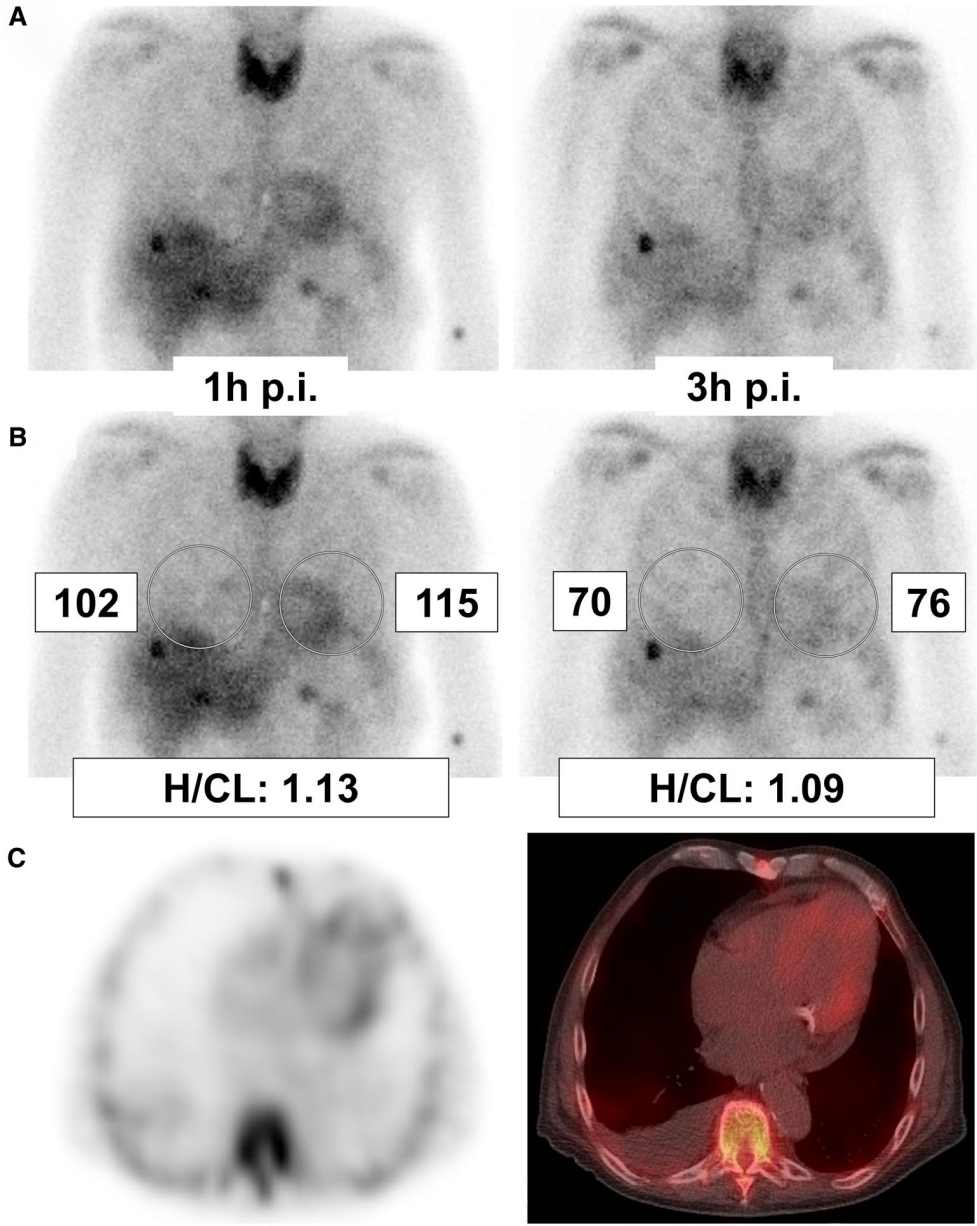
Patient example. 79-year-old male with proven AL amyloidosis. Planar anterior images at 1 hour and 3 hour p.i. are displayed (A raw images; B with regions of interest [ROIs], mean counts and H/CL ratios). Both time points show pathological uptake in the thyroid gland and liver due to AL amyloidosis. At 1 hour p.i. (A left), blood pool-related activity in the heart led to a visual score of 2 (i.e., false positive). At 3 hour p.i. (A right), visual assessment was impaired by hepatic uptake; the visual score was 1 (true negative). SPECT and SPECT/CT (C) confirmed mild myocardial uptake, which can be observed in AL amyloidosis. In contrast to visual assessment, H/CL ratios were unequivocally true negative at both time points, and despite the different visual appearance of the heart, H/CL ratio was only marginally higher at 1 hour than at 3 hour p.i. (B). This may be explained by the simultaneous increase of counts in the right hemithorax ROI resulting from increased blood pool at 1 hour p.i., which is disregarded during visual assessment
